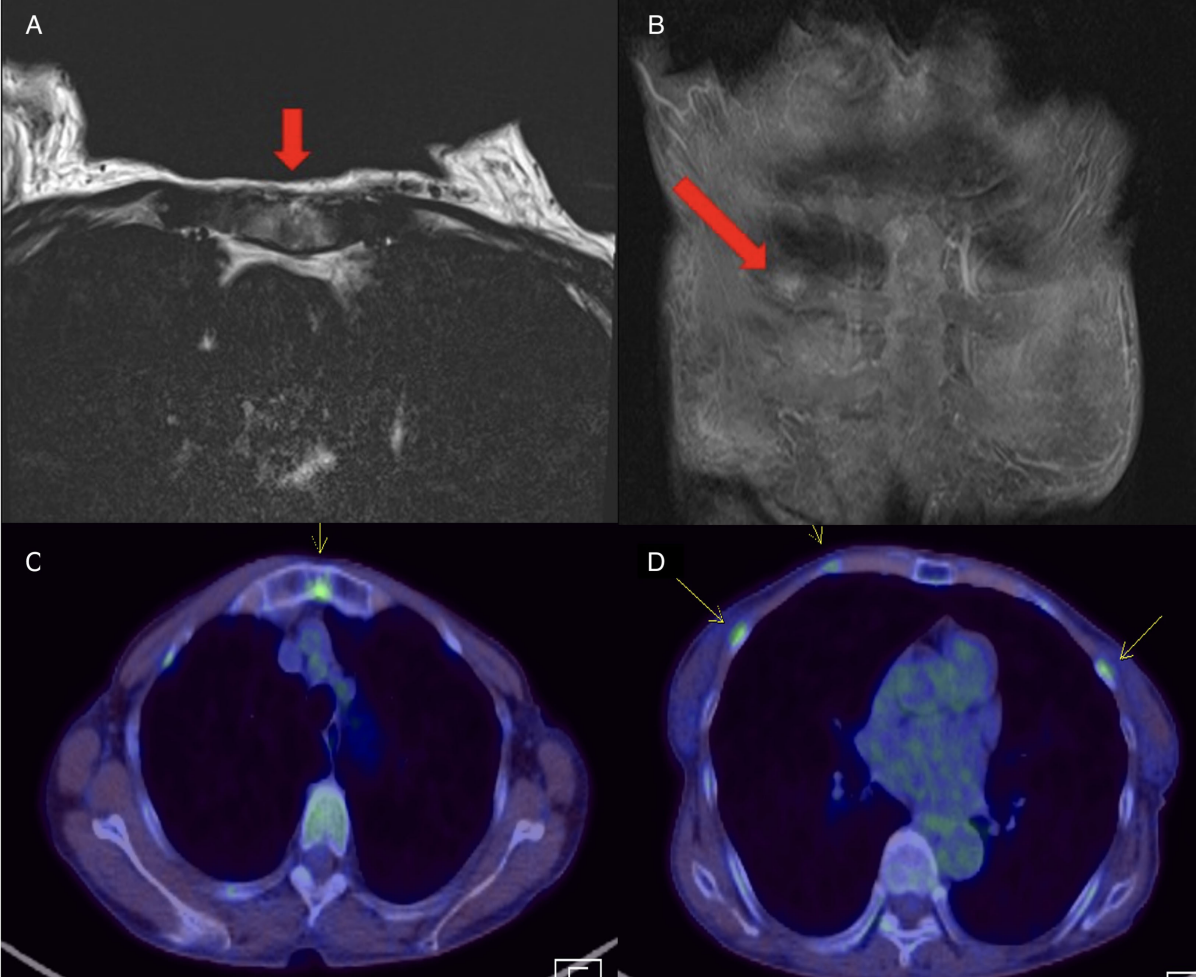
(A, B) Chest MRI scans. Red arrows indicate high-signal-intensity lesions at the sternum and right third rib. (C, D) PET-CT scans. Yellow arrows indicate multiple FDG uptakes in the sternum and ribs. High-signal-intensity lesions on MRI were consistent with lesions with FDG uptake on PET-CT. MRI, magnetic resonance imaging; PET-CT, positron emission tomography–computed tomography; FDG, fluorodeoxyglucose.
